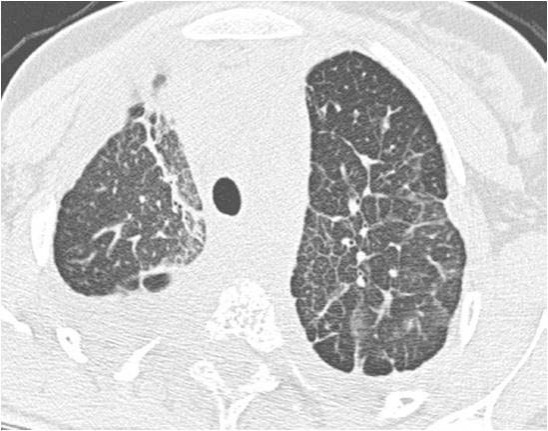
Lung CT scan (case #8) showing lymphangiectasies.
